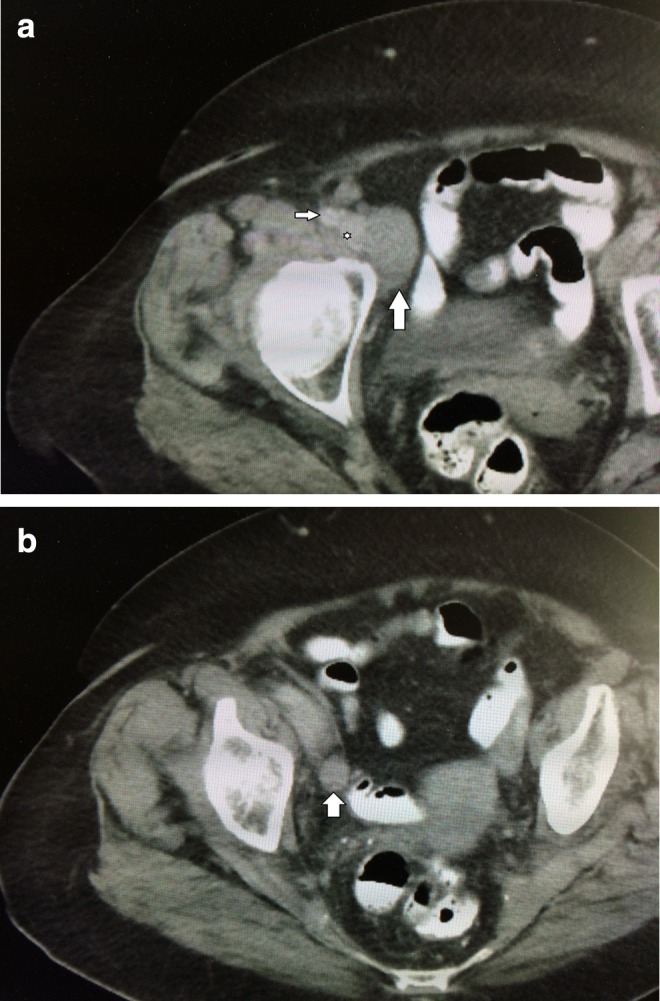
(a) CT scan demonstrating 3.3 cm right external iliac node (*vertical arrow*), right external iliac vein (*asterisk*), and right external iliac artery (*horizontal arrow*). (b) CT scan showing 3.2 cm right obturator lymph node enlargement (*vertical arrow*).
